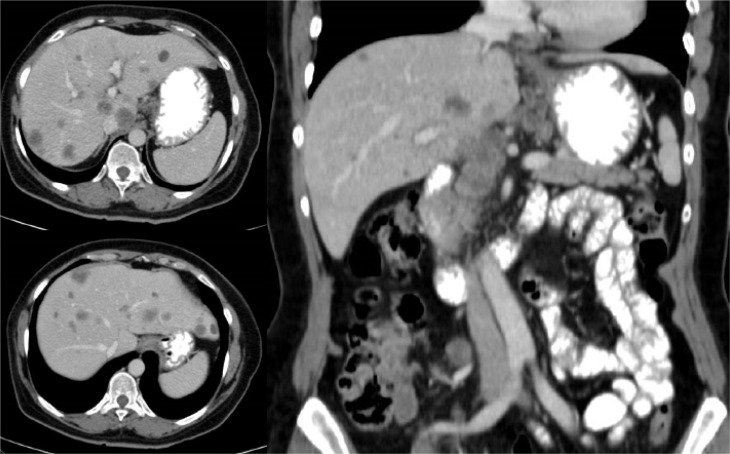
Basal CT scan. Multiple and bilobar hepatic metastases are observed
